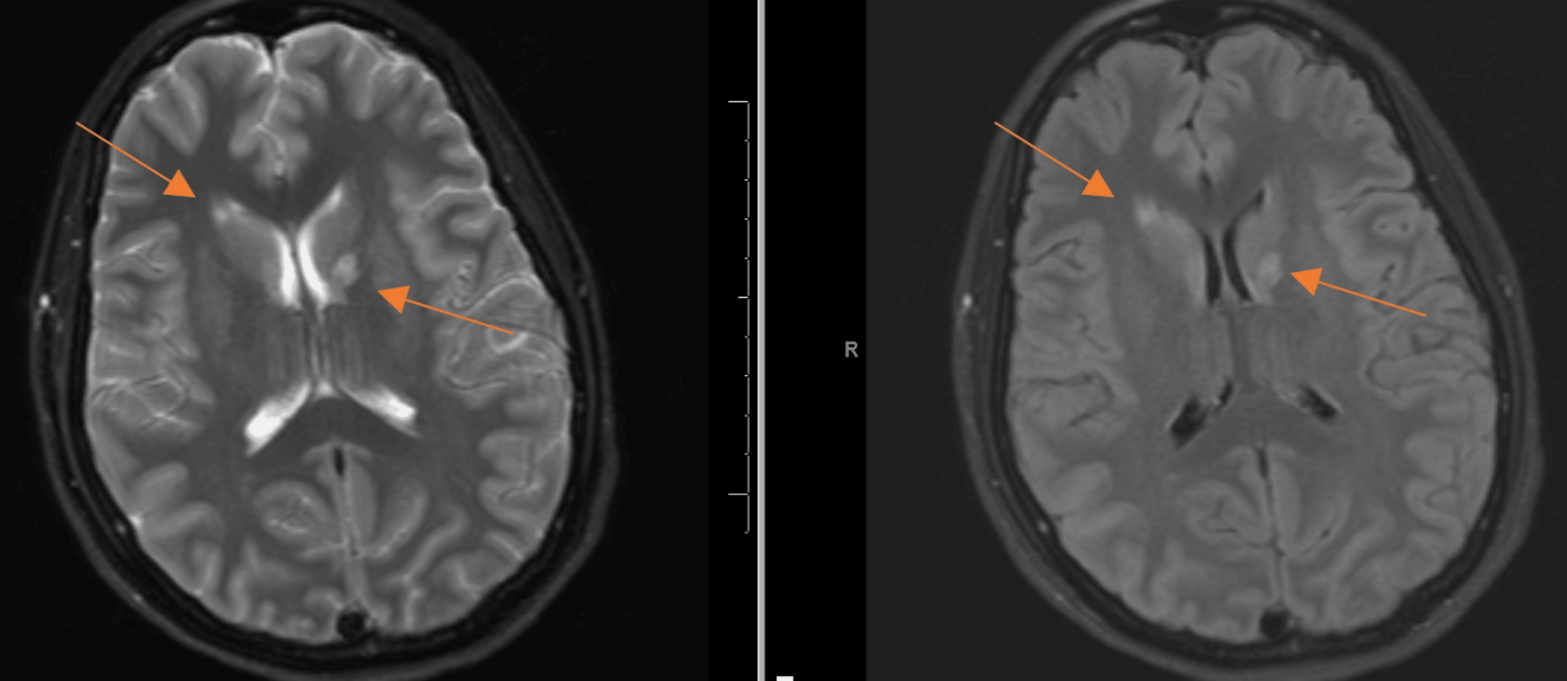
Brain MRI with and without contrast at the initial presentation demonstrating T2 FLAIR hyperintensities in the bilateral ganglio-capsular structures and surrounding white matter, with additional involvement of the left thalamus and the genu of the corpus callosum (arrows) MRI: magnetic resonance imaging, FLAIR: fluid-attenuated inversion recovery
